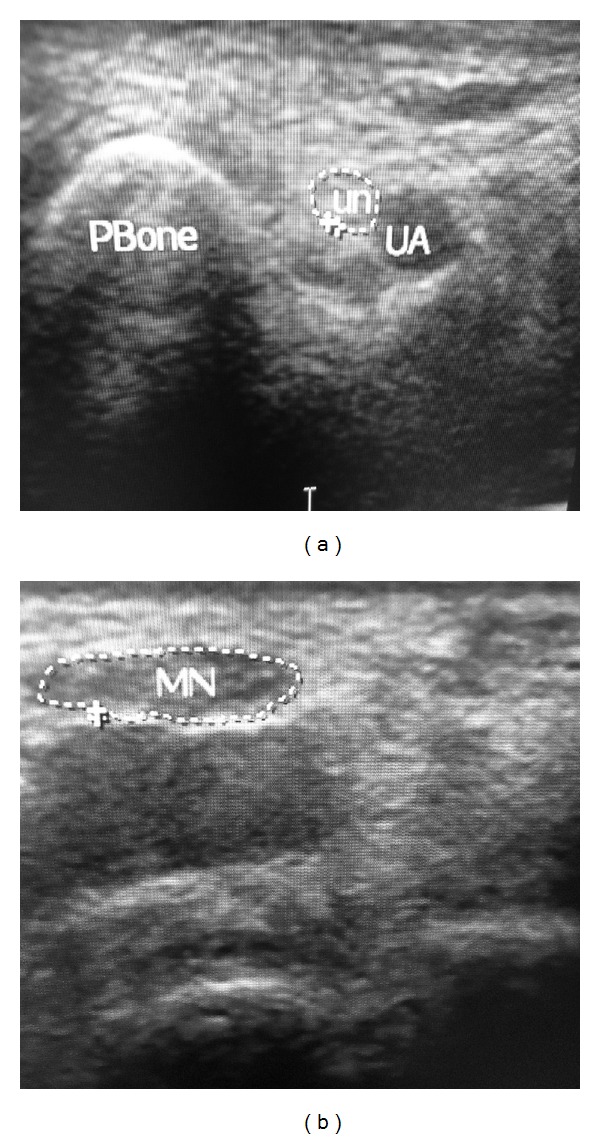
Wrist ultrasound at the carpal tunnel inlet for patient with mild CTS: (a) medial and (b) lateral aspects of the wrist (the tracing method for measuring the cross sectional area of the median nerve which was 10 mm^2^). UA: ulnar artery, UN: ulnar nerve in the Guyon canal, Pbone: pisiform bone, MN: median nerve.
